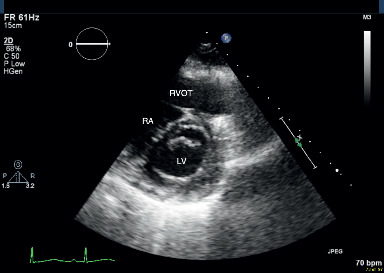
Parasternal short-axis view at the mitral valve level, performed on the day following thrombolysis. Note the absence of both the thrombus and the D-shaped interventricular septum. RA: right atrium; RVOT: right ventricular outflow tract; LV: left ventricle.
